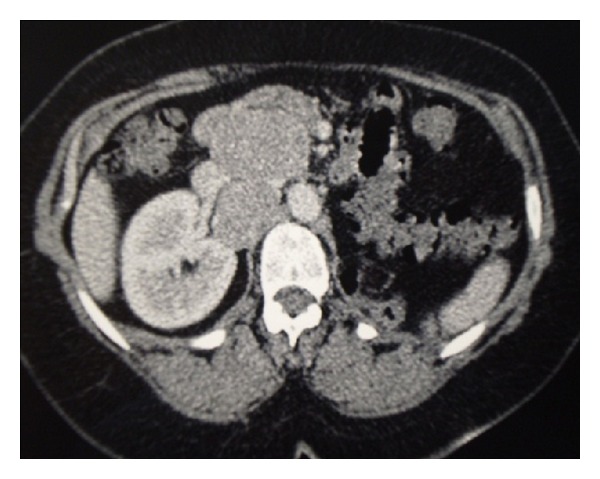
Contrast enhanced abdominal CT scan shows an empty renal lodge on the left side with voluminous retroperitoneal lymphadenopathies pre- and retroinferior cava rolling the inferior vena cava and the right renal vein.
